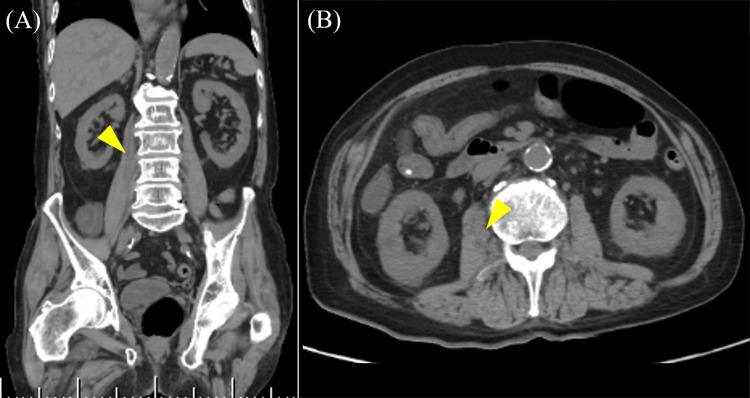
Abdominal CT imaging on day 59. Abdominal CT showing hypertrophy of the right iliopsoas muscle and a low-density area inside the right iliopsoas muscle. (A) Coronal section. (B) Axial section.
